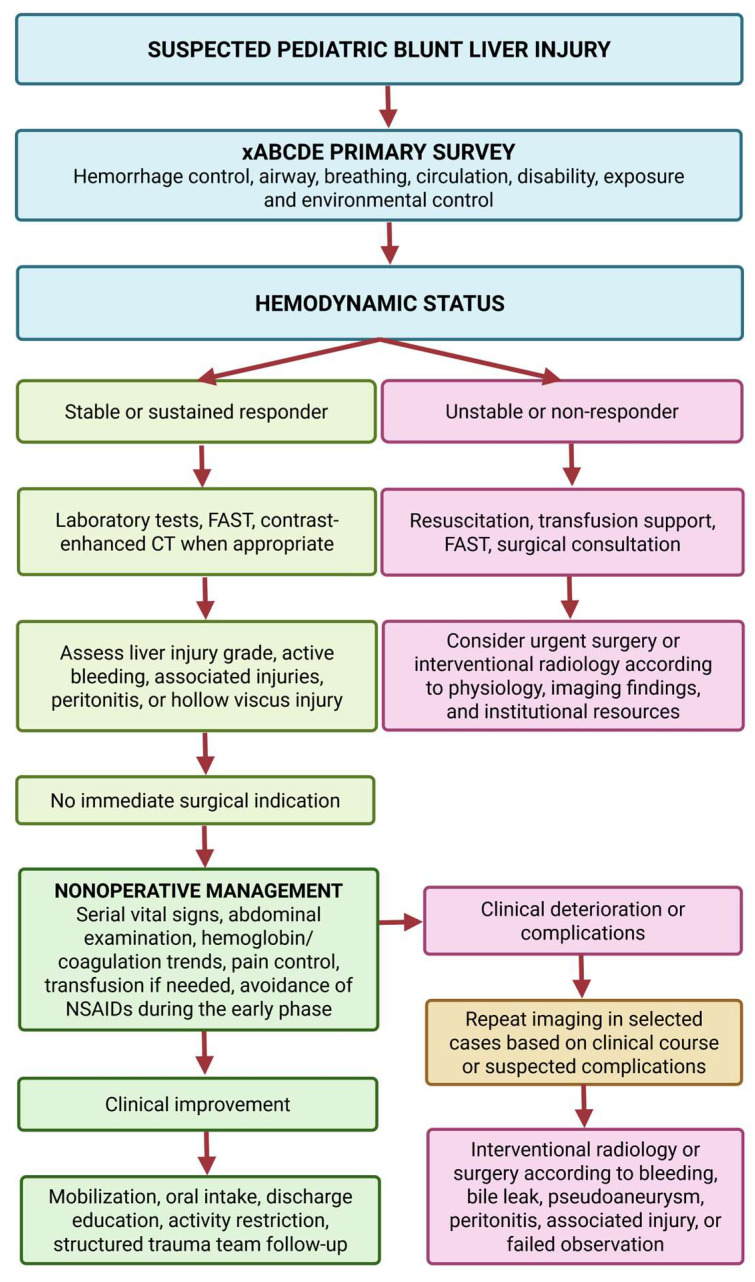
Proposed Assessment and Management Algorithm for Pediatric Blunt Liver Injury. This figure summarizes a proposed clinical pathway for suspected pediatric blunt liver injury, developed by the authors based on the reviewed literature and current pediatric trauma management principles. The algorithm begins with the xABCDE primary survey, including immediate control of exsanguinating hemorrhage when present, followed by assessment of airway, breathing, circulation, disability, exposure and environmental control, vital signs, and physical examination. Hemodynamic status guides the early pathway. Stable patients or those with a sustained response to resuscitation undergo laboratory testing, FAST, and contrast-enhanced CT when appropriate, followed by evaluation of injury grade, active bleeding, and associated lesions. Patients without immediate indications for surgery may proceed to nonoperative management with structured clinical, hemodynamic, and laboratory reassessment, pain control, transfusion if needed, and avoidance of nonsteroidal anti-inflammatory drugs (NSAIDs) during the early phase. Repeat imaging should be reserved for selected cases based on the clinical course or suspected complications. Escalation to interventional radiology or surgery should be guided by patient physiology, local expertise, and the suspected mechanism of failure, including hemorrhagic, biliary, vascular, or peritoneal complications, as well as complications related to associated injuries.
